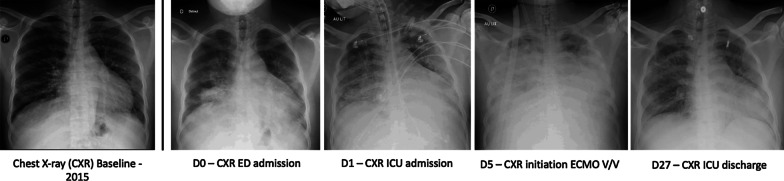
Chest X-ray (CXR) evolution through hospitalization
